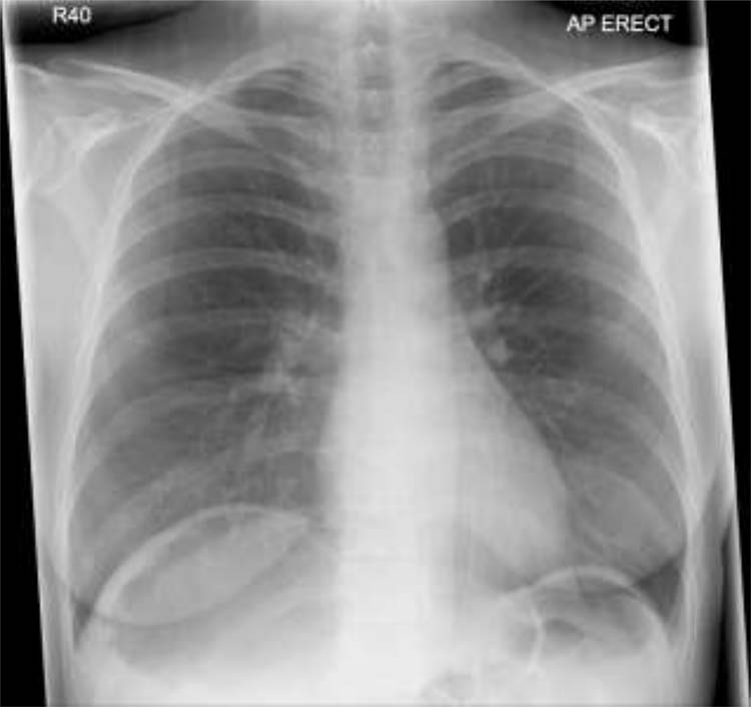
Chest X-ray shows air under diaphragm due to Chilaiditi syndrome.
